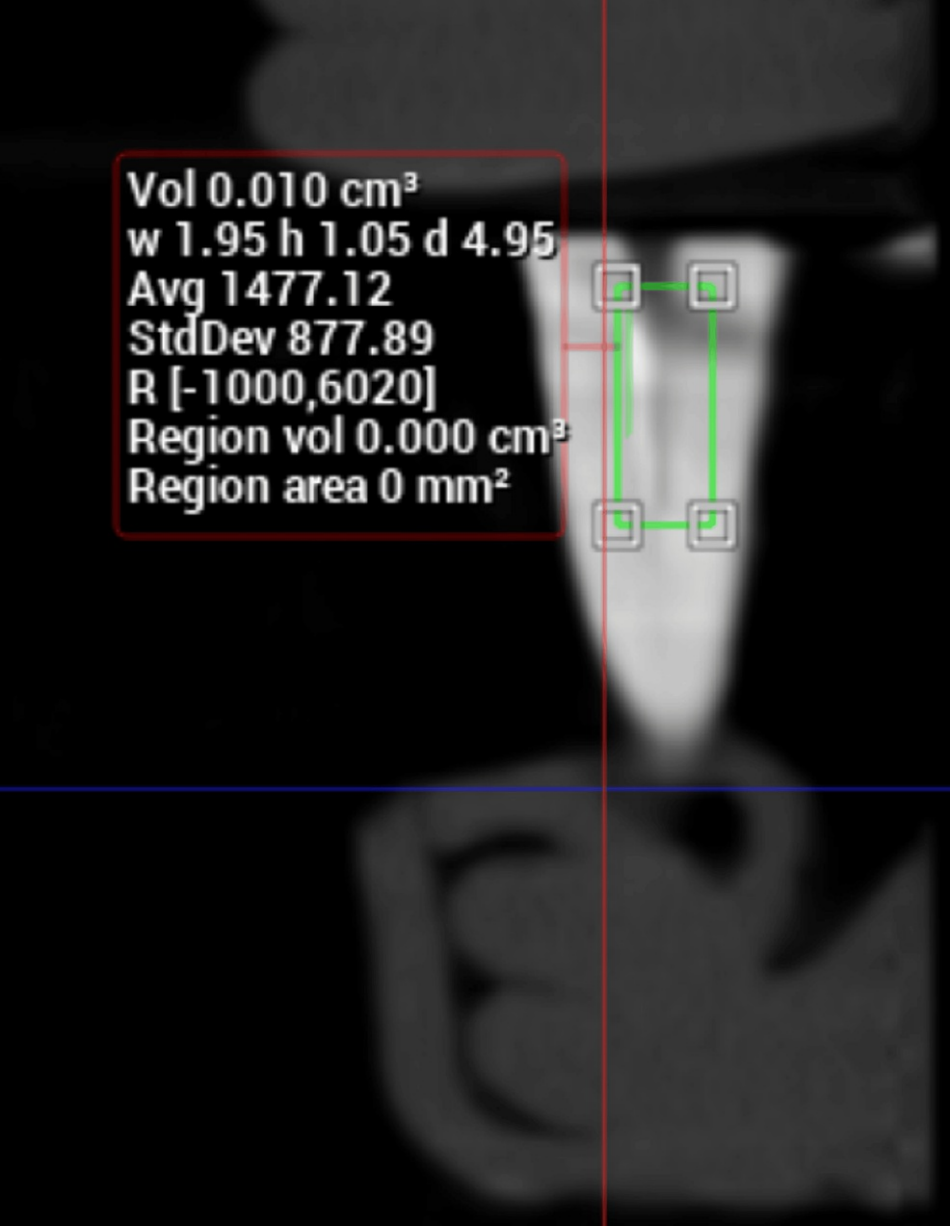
Calculation of volume from CBCT image after supplementary step CBCT: Cone beam computed tomography
